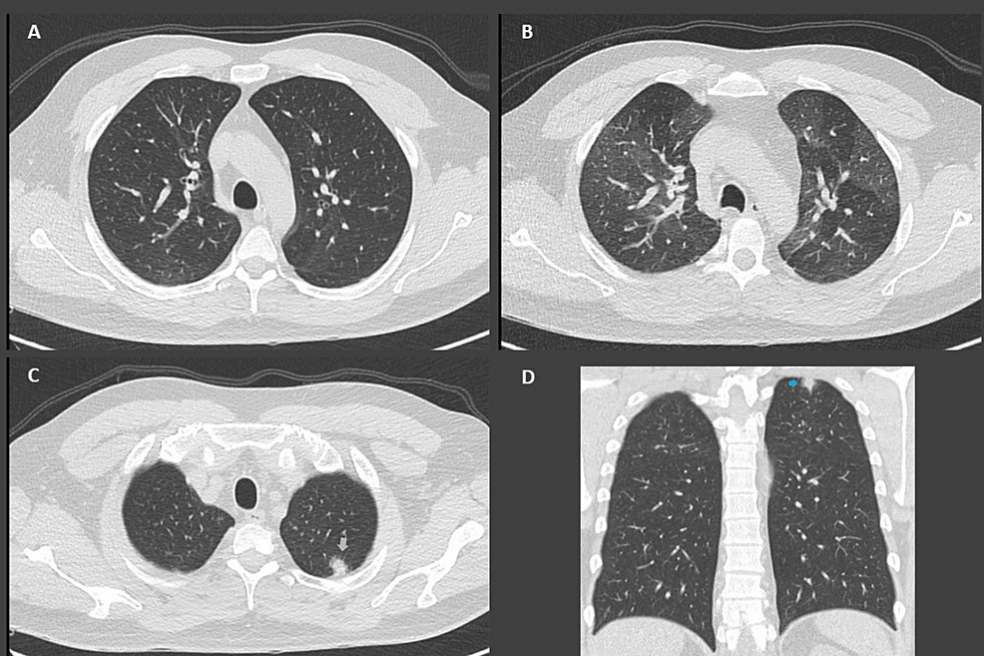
CT scan for Patient 1 A: The CT scan shows lung slides during the inspiratory phase. B: shows mosaic attenuation pattern on expiratory phase slides. C-D: A nodule in the apico-posterior segment of the left upper lobe (yellow arrow) surrounded by a ground-glass zone (blue arrow)
